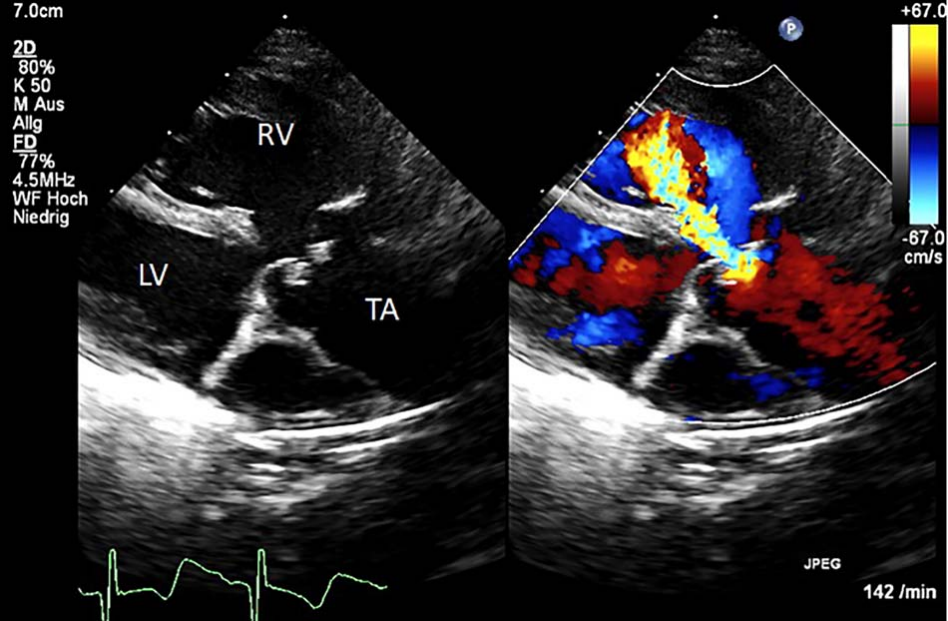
Color Doppler echocardiography in the parasternal long axis view shows severe TV regurgitation. RV: right ventricle, LV: left ventricle, TA: truncus arteriosus.
